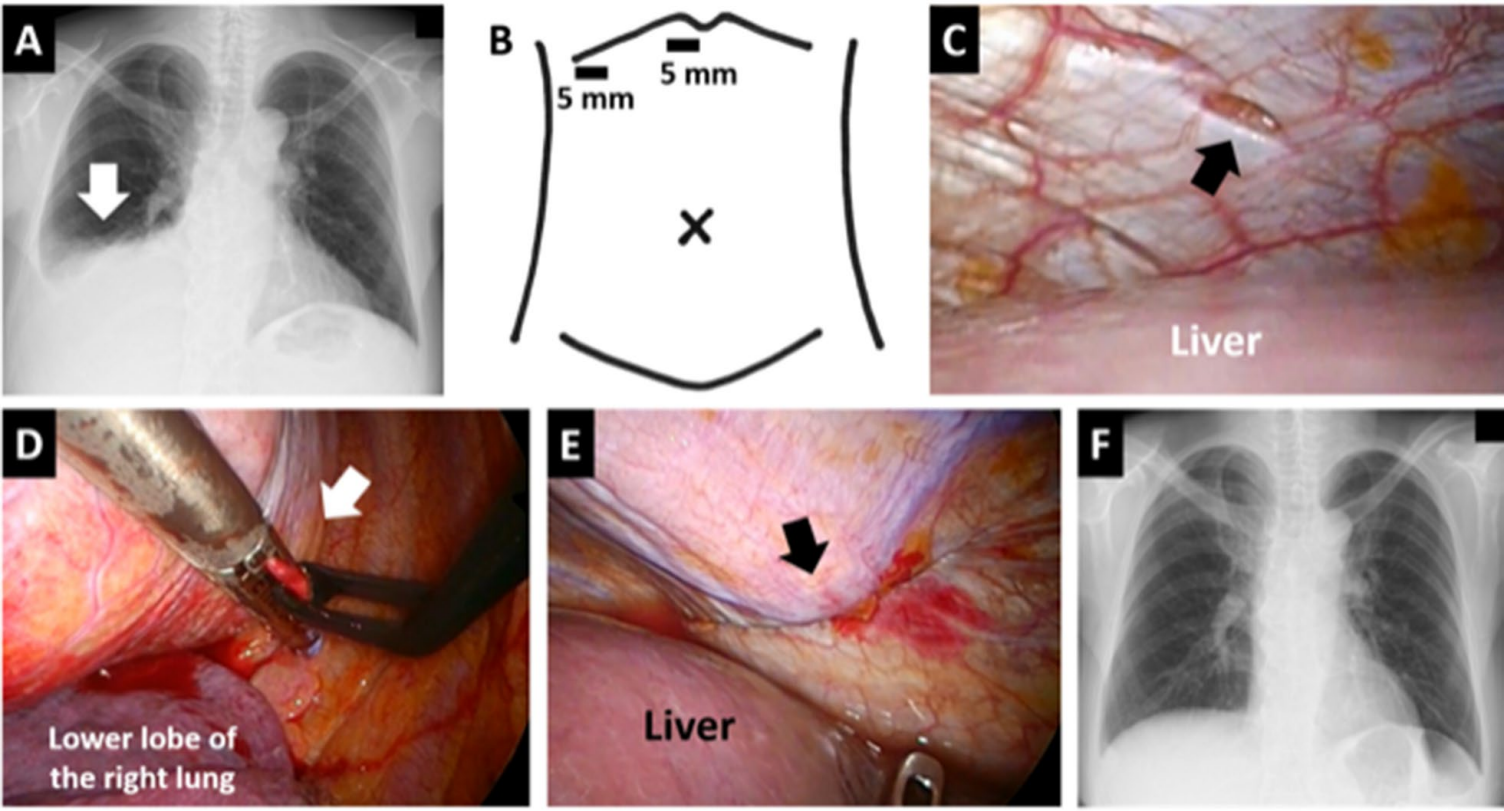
Diagnosis and treatment for pleuroperitoneal communication in Patient 1. A Chest radiograph taken 2 months after the introduction of peritoneal dialysis. An arrow shows right pleural effusion. B. Port positions in the laparoscopic approach. C Laparoscopic view of the diaphragmatic fistula. An arrow shows a 2-mm-sized fissure in the central tendon of the right diaphragm over the liver. D A thoracic view of the closure of the diaphragmatic fistula using an automatic suture device. An arrow shows the grasped site of the right diaphragm, including the fistula. E Laparoscopic view of the closed site. The arrow shows the suture line. F Chest radiograph taken 3 months after the surgery
